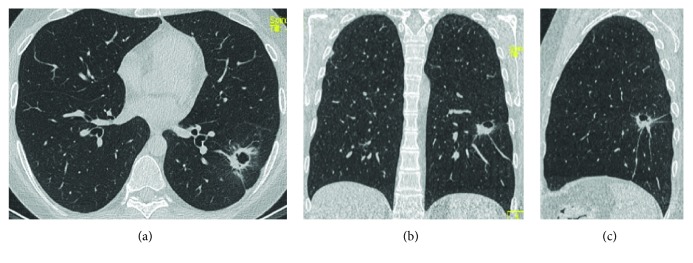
A 55-year-old woman with coexisting CD and GPA disease. These HRCT images (axial (a), coronal (b), sagittal (c)) show a pulmonary necrotic nodule in the lower left lobe: it is possible to notice the necrotic cavity inside. Whether this is due to CD of GPA was unclear.
